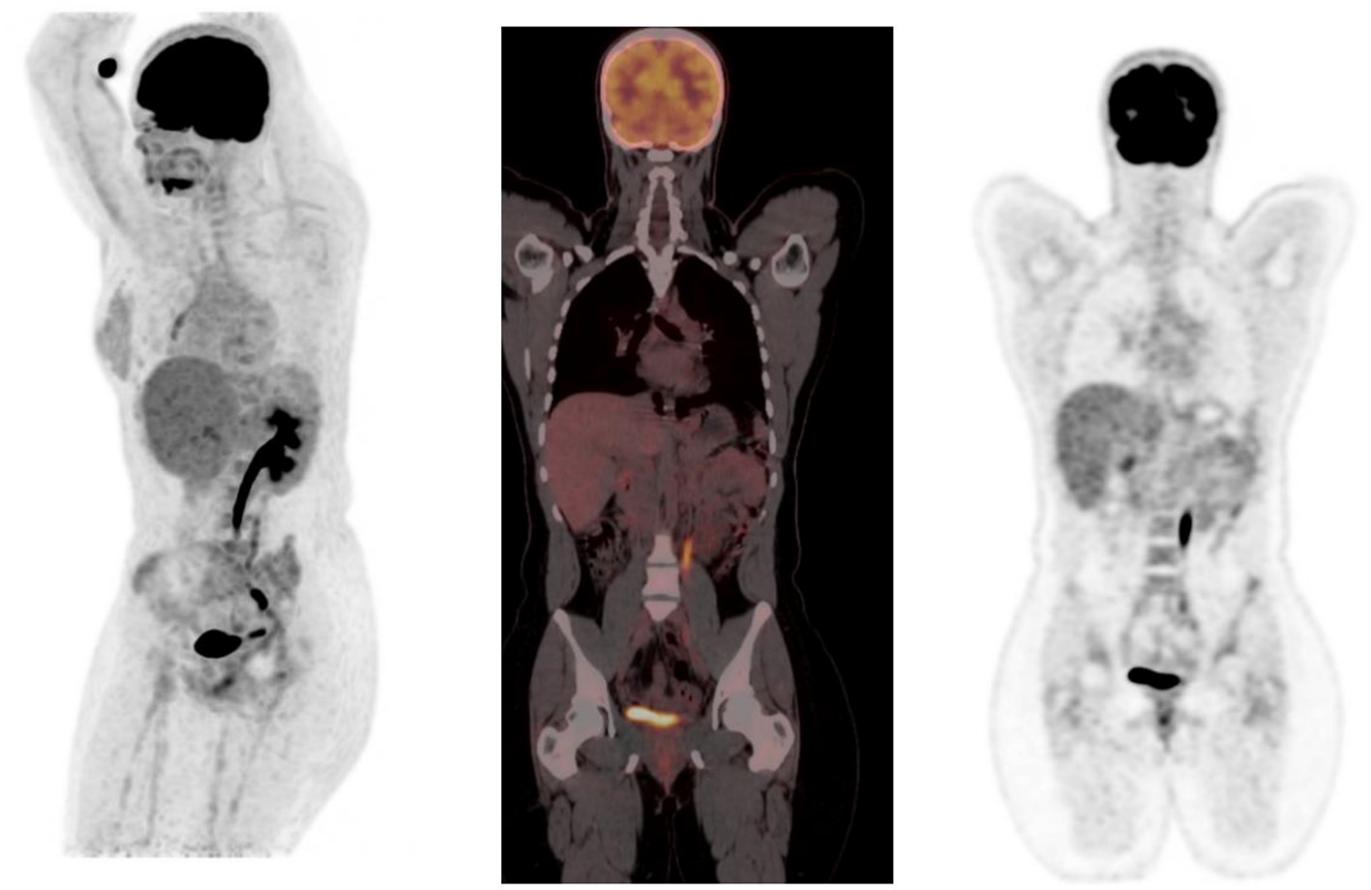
MIP and coronal FDG PET/CT and PET images showing physiological activity in the left renal collecting system, left ureter and urinary bladder. The MIP image is helpful in differentiating tracer excretion in the ureter from nodal pathology. Identifying the uptake to the ureter on CT also helps in differentiating from nodal pathology.
